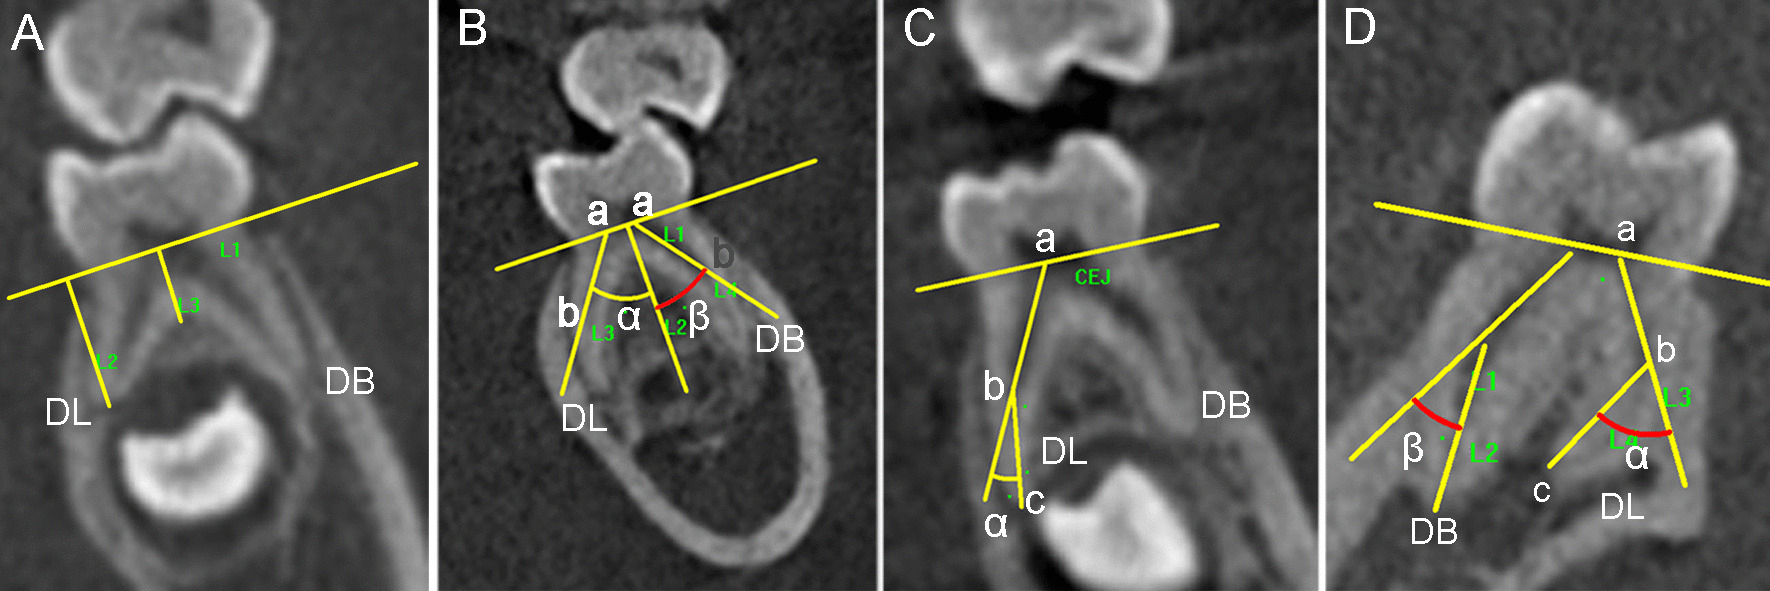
Odontometric measurement of the DB and DL roots of three-rooted deciduous mandibular second molars (DMSMs) and a three-rooted permanent mandibular first molar (PMFM) in the CBCT images (from the adjusted proximal view). a L2 is the vertical length of the DL root; L3 is the level of the distal root furcation (a DMSM). b Angle α is the spreading angle of DL root, and angle β is the spreading angle of DB root (a DMSM). c Angle α is the curvature angle of the DL root measured by Schneider’s method (a DMSM). d Measurement of the angle of root curvature of the DL and DB root by Schneider’s method (a PMFM)
